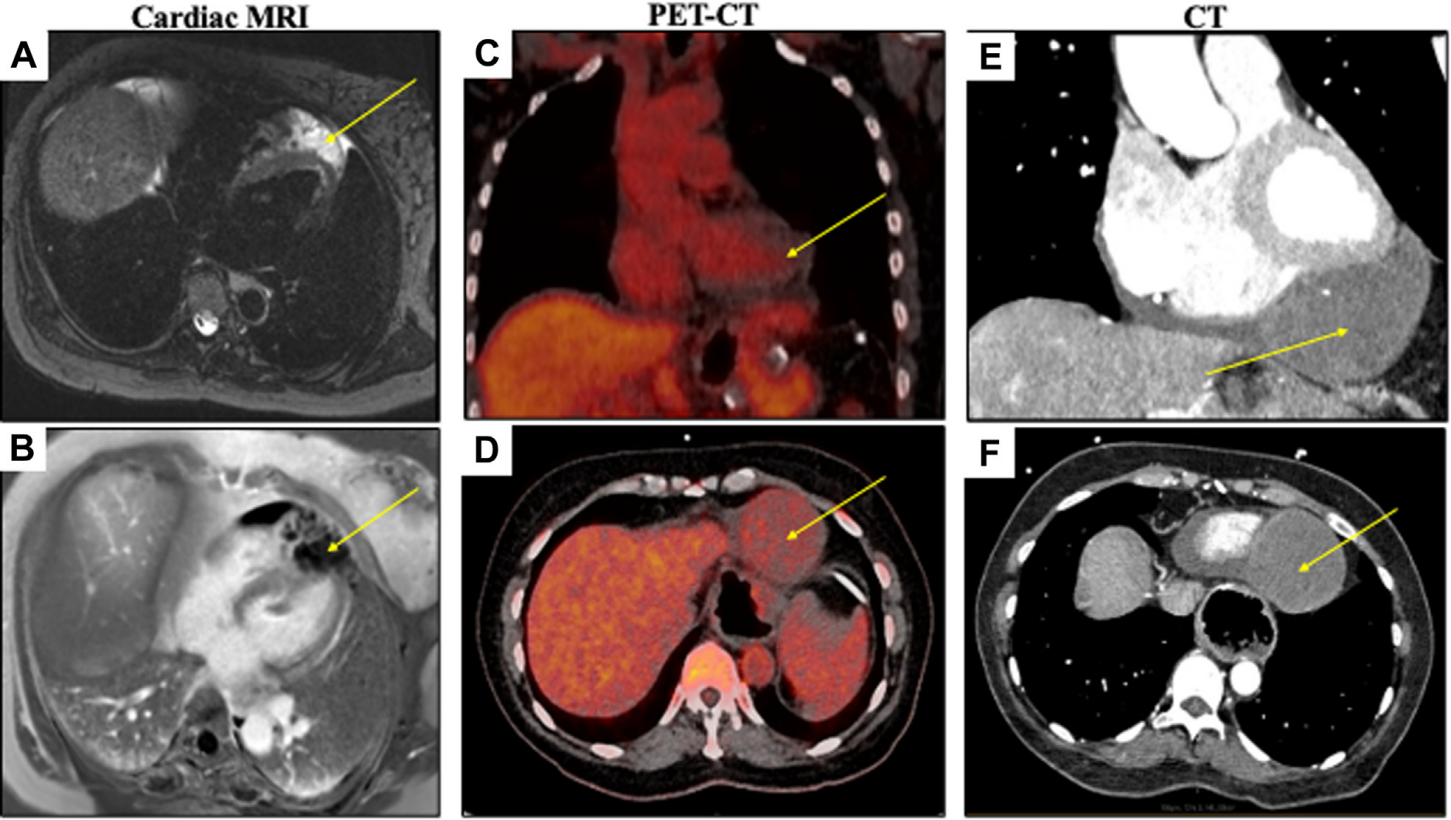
Multimodality Imaging Cardiac magnetic resonance, positron emission tomography (PET), and computed tomography (CT) are shown. (A) Cardiac MRI with T2 signaling and (B) late gadolinium enhancement showing a 7.7 × 5.7 cm well-circumscribed, multiseptated, heterogeneously enhancing mass in the pericardial space, inferior to the left ventricular apex. (C, D) PET-CT showing a 6.0 × 6.4 × 7.8 cm solid mass arising from the left pericardial space demonstrating low-level radiotracer uptake. (E, F) Coronal and axial CT images show a 7.0 × 6.9 × 4.2 cm intrapericardial mass abutting the inferior left ventricular wall and apex and encasing the distal left anterior descending artery.
